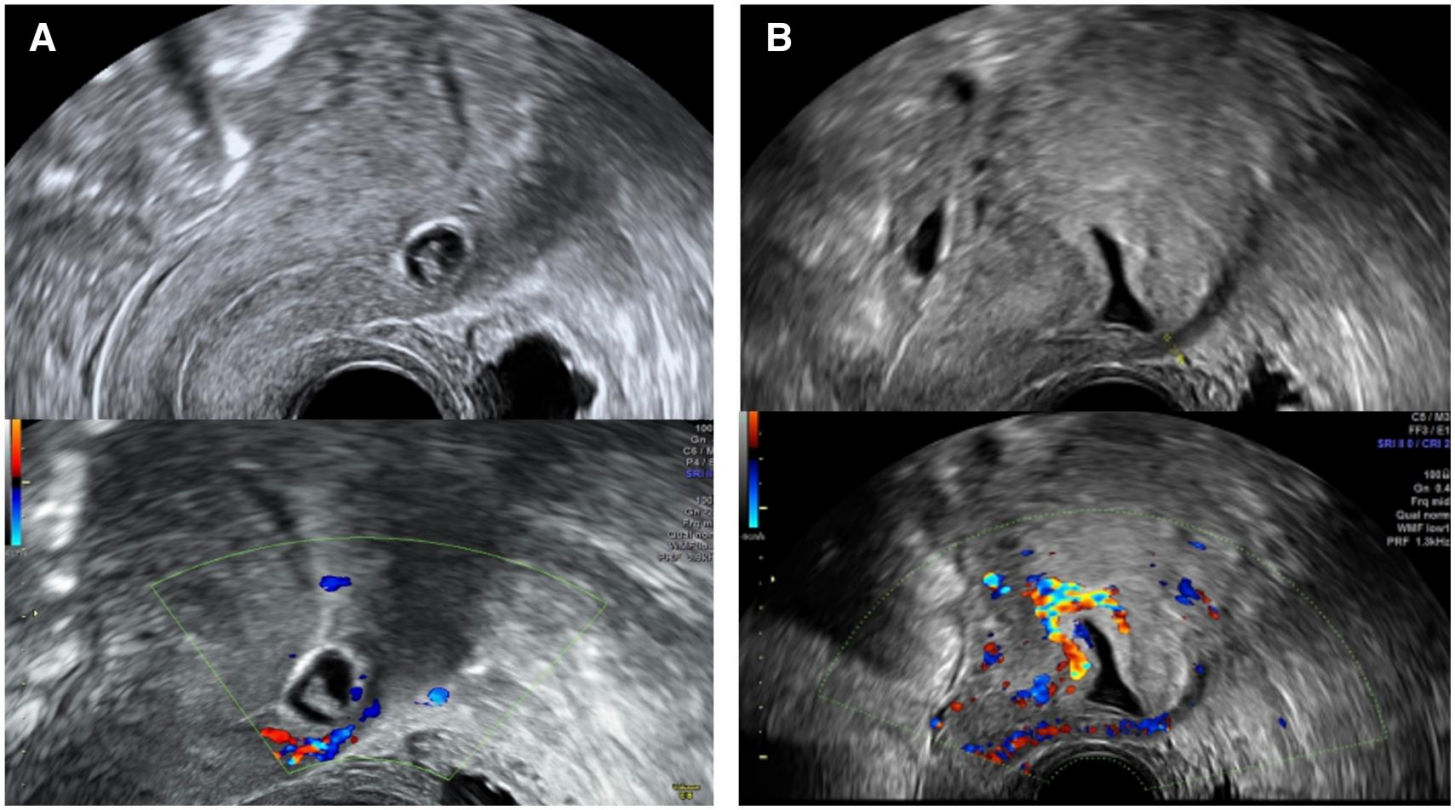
Ultrasound images comparing true Caesarean scar ectopic pregnancy (CSEP) to eutopic pregnancy with posterior implantation. These images originate from the same patient in two consecutive pregnancies after a Caesarean section. In (A), a true CSEP, the pregnancy is within the niche and blood supply is derived from the anterior uterine wall. In (B), a eutopic pregnancy with posterior implantation, Doppler examination reveals that the pregnancy is posteriorly implanted, and therefore does not represent a CSEP.
